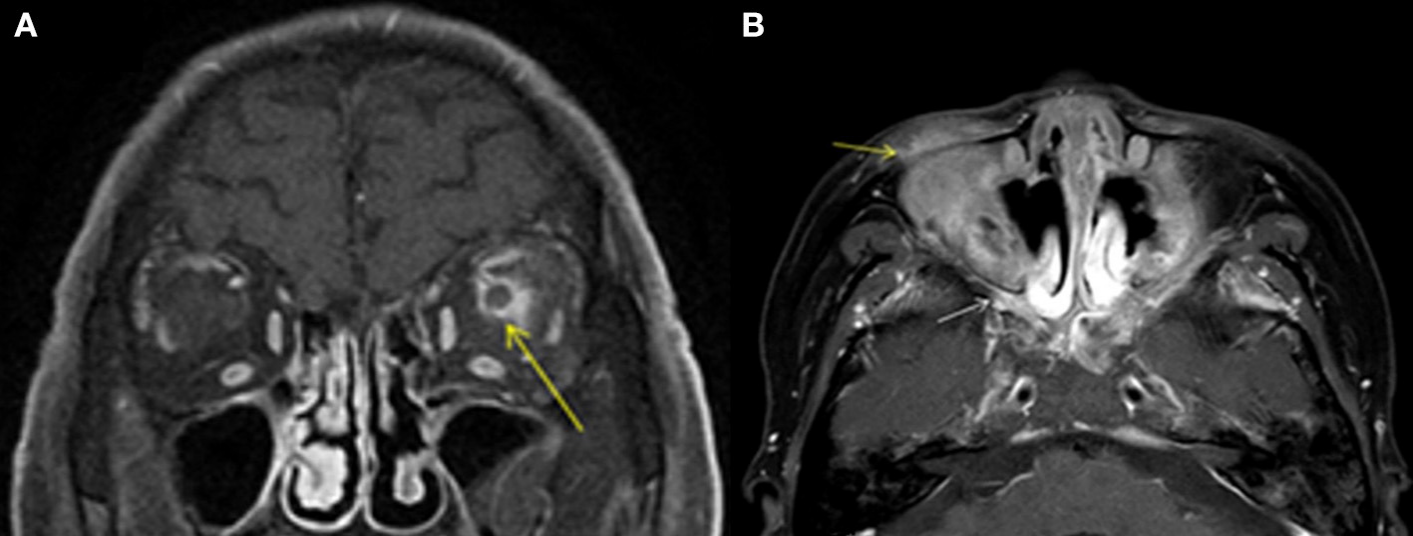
MRI findings of OPN patients with GAP. (A) Coronal contrast enhanced T1-weighted MRI showing thickening and enhancement of the left optic nerve sheath (arrow). (B) Axial contrast enhanced T1-weighted MRI showing abnormal contrast enhancement shadow in the bilateral pterygopalatine fossa (white arrow) and inflammatory infiltration in the eyelid (yellow arrow).
